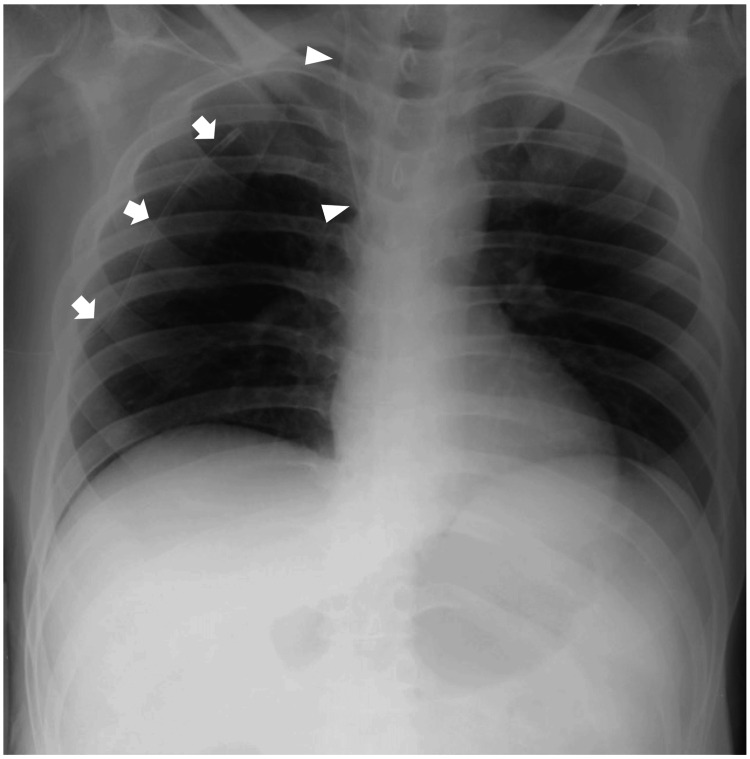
A plain film chest radiograph following chest drain insertion obtained on day 2 of hospitalization The right lung is re-expanded with no evidence of pneumothorax or overt pulmonary congestion. The cardiac silhouette is normal. The arrows indicate a chest drain appropriately positioned with its tip pointing superiorly within the pleural cavity. The arrowheads indicate a central venous catheter inserted via the right internal jugular vein, with its tip placed in the superior vena cava or at the cavoatrial junction.
